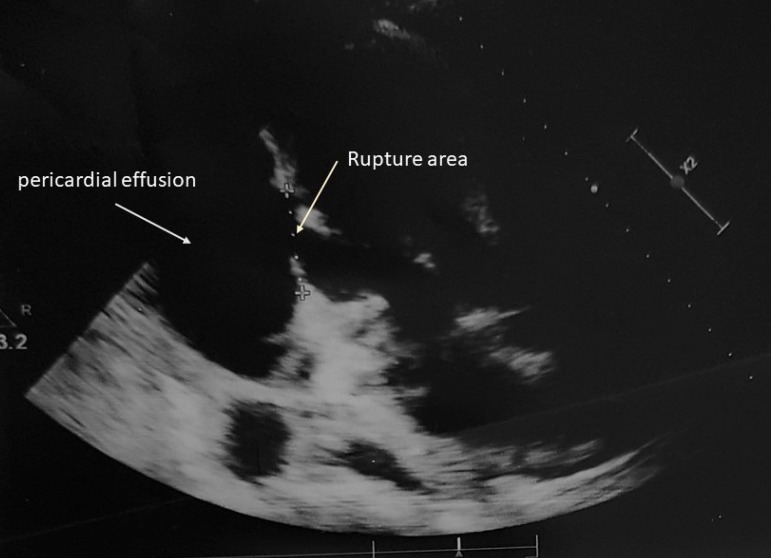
Echocardiogram showing the myocardial rupture area and pericardial effusion.
